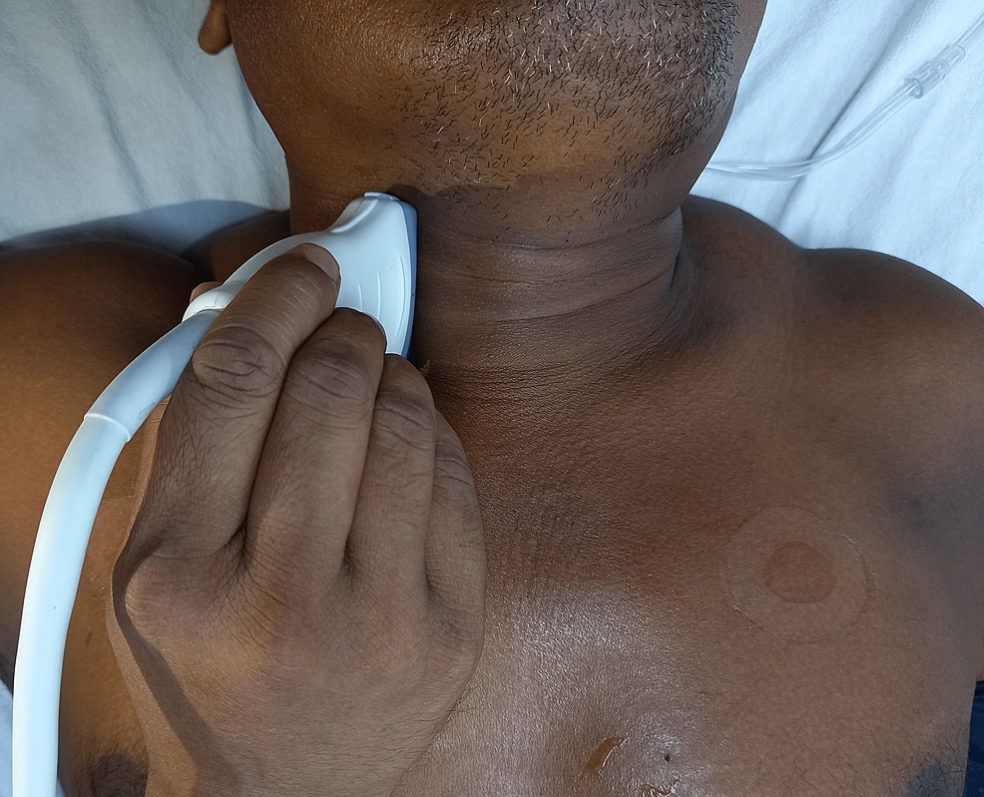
Probe position for carotid Doppler measurements (anterior view)
